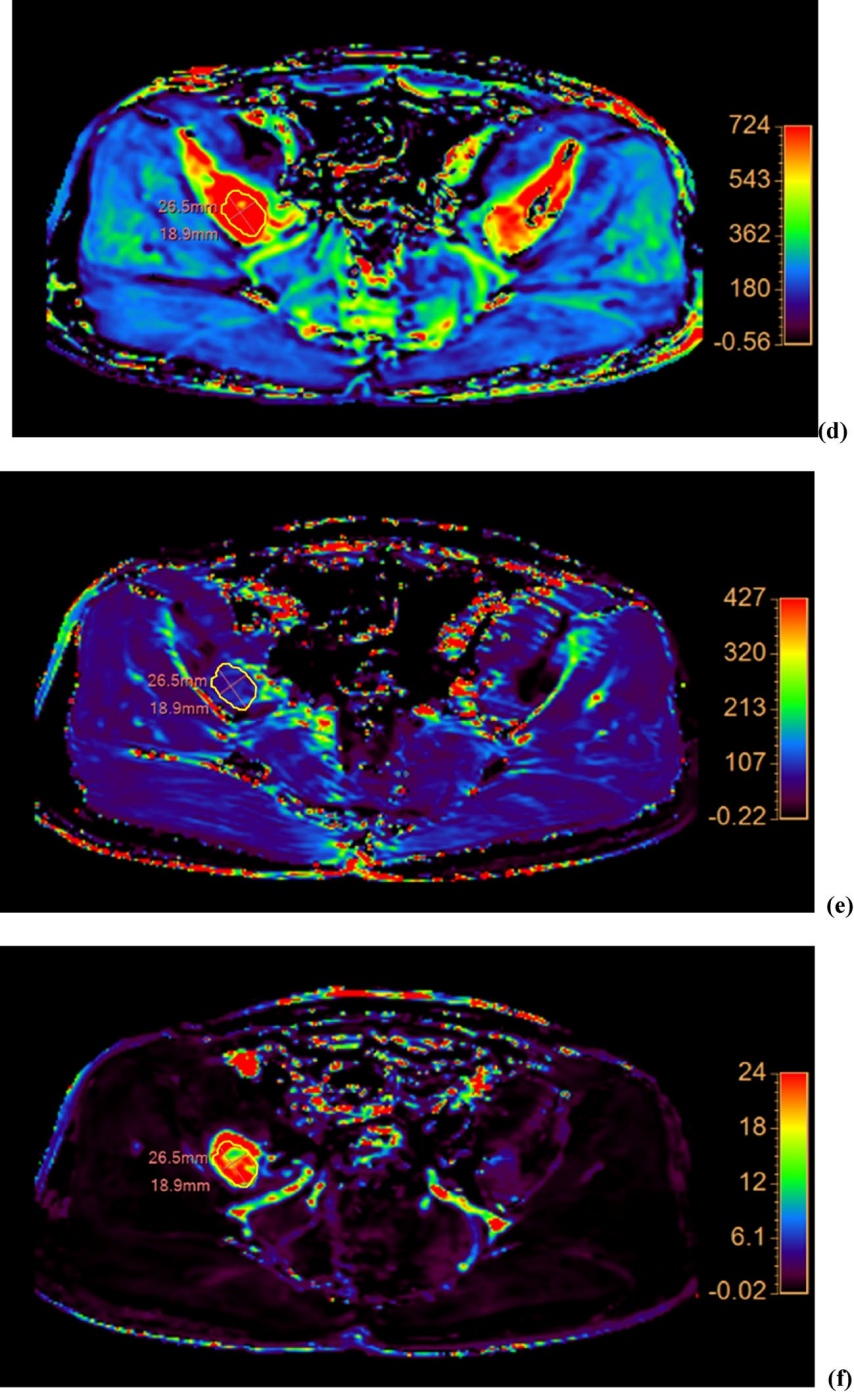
Images of a 64-year-old man with hepatocellular carcinoma showing metastasis to the right iliac bone. (a) The ROI was drawn within the lesion encompassing the whole tumor volume on axial T1-weighted images and reformatted coronal (right upper panel) and sagittal (right lower panel) images. The corresponding ADC map (b) and color-encoded overlay maps of the *K*^trans^ (c), *k*_ep_ (d), *v*_*e*_ (e), and *v*_*p*_ (f) are shown with ROIs and scale bars. ADC, apparent diffusion coefficient; *K*^trans^, volume transfer constant; *k*_ep_, rate constant; *v*_*e*_, volume fraction of the extravascular extracellular matrix; *v*_*p*_, blood plasma volume; ROI, region of interest.
